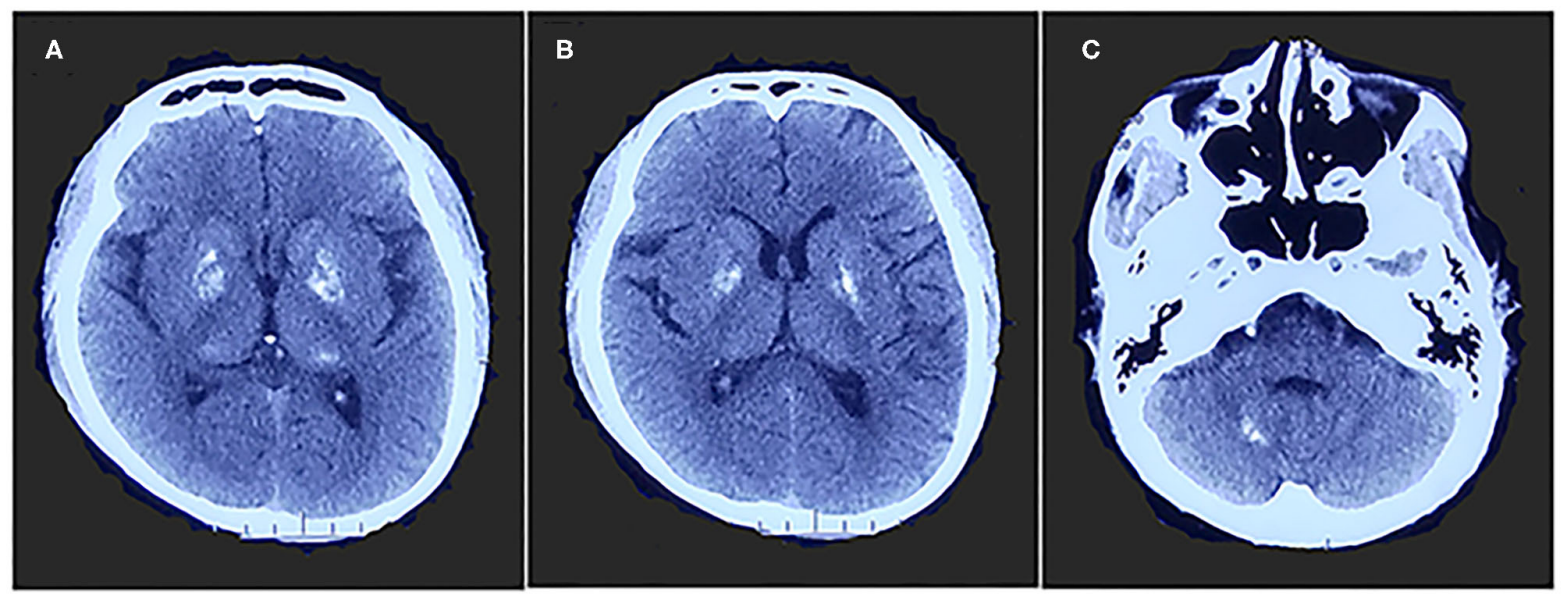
Brain computed tomography shows calcifications of bilateral basal ganglia, thalamus (A,B), and cerebellar dentate nuclei (C).
